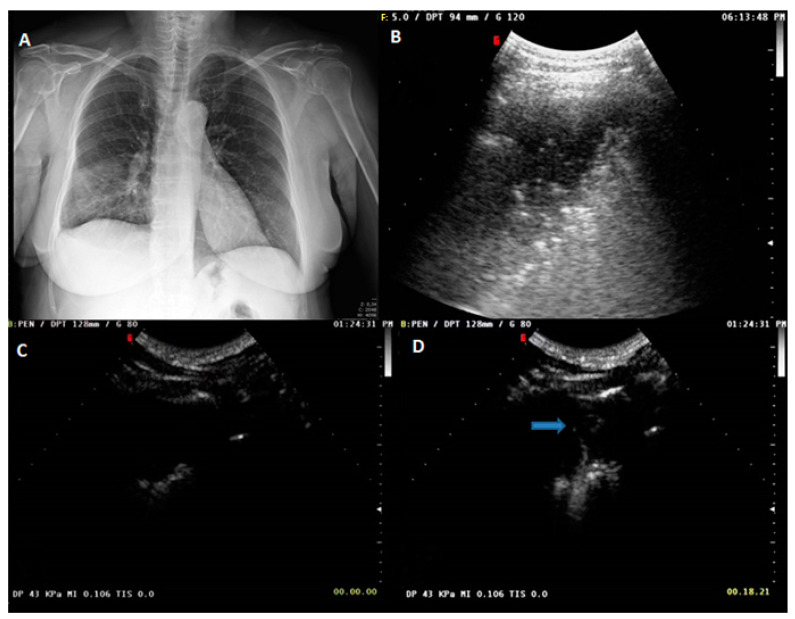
(A) Chest radiograph showing ill-defined patchy consolidation and ground glass opacities (GGO) in the right middle to lower lung zone. The clinical–radiological diagnosis was pneumonia. (B) US scan (thoracic pre-setting) in B-mode showing a hypoechoic subpleural pulmonary lesion with inner hyperechoic spots. (C) CEUS scan with SonoVue at 0 s showing no lesion perfusion. (D) CEUS scan with SonoVue at 18 s showing first spots of arrival of the ultrasound contrast agent (blue arrow).
